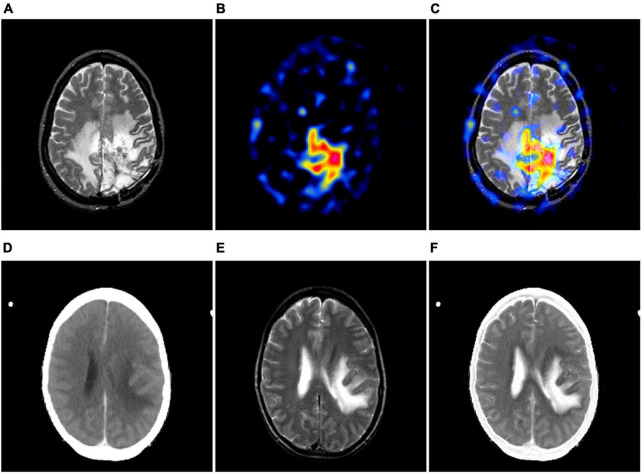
The examples of multimodal medical images. (A, B) are MR-T2-weighted image and the PET image of the same case; (D, E) are CT and MR-T2-weighted image of the same case. (C, F) show its corresponding image fusion results, respectively.
